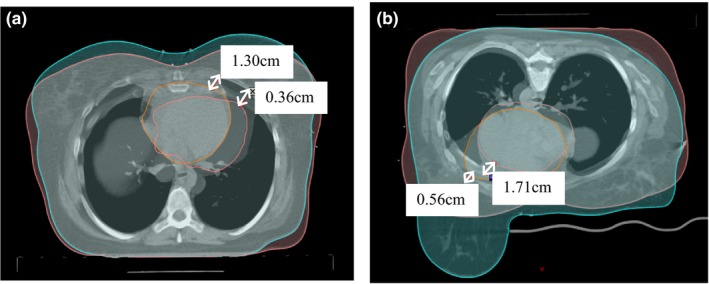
(a) Image fusion of the CT scans in the Free Supine versus the DIBH position. The supine position is in pink, and the DIBH position is in blue. For the supine position, the heart‐to‐chest distance was 0.36 cm, and this distance increased to 1.30 cm when the patient was positioned in DIBH. (b) Image fusion of the CT scans in the Free Supine versus the prone position. The supine position is in pink, and the prone position is in blue. For the supine position, the heart‐to‐chest distance was 1.71 cm, and this distance decreased to 0.56 cm when the patient was positioned in prone. The supine scan was rotated 180 degrees to align with the prone scan.
